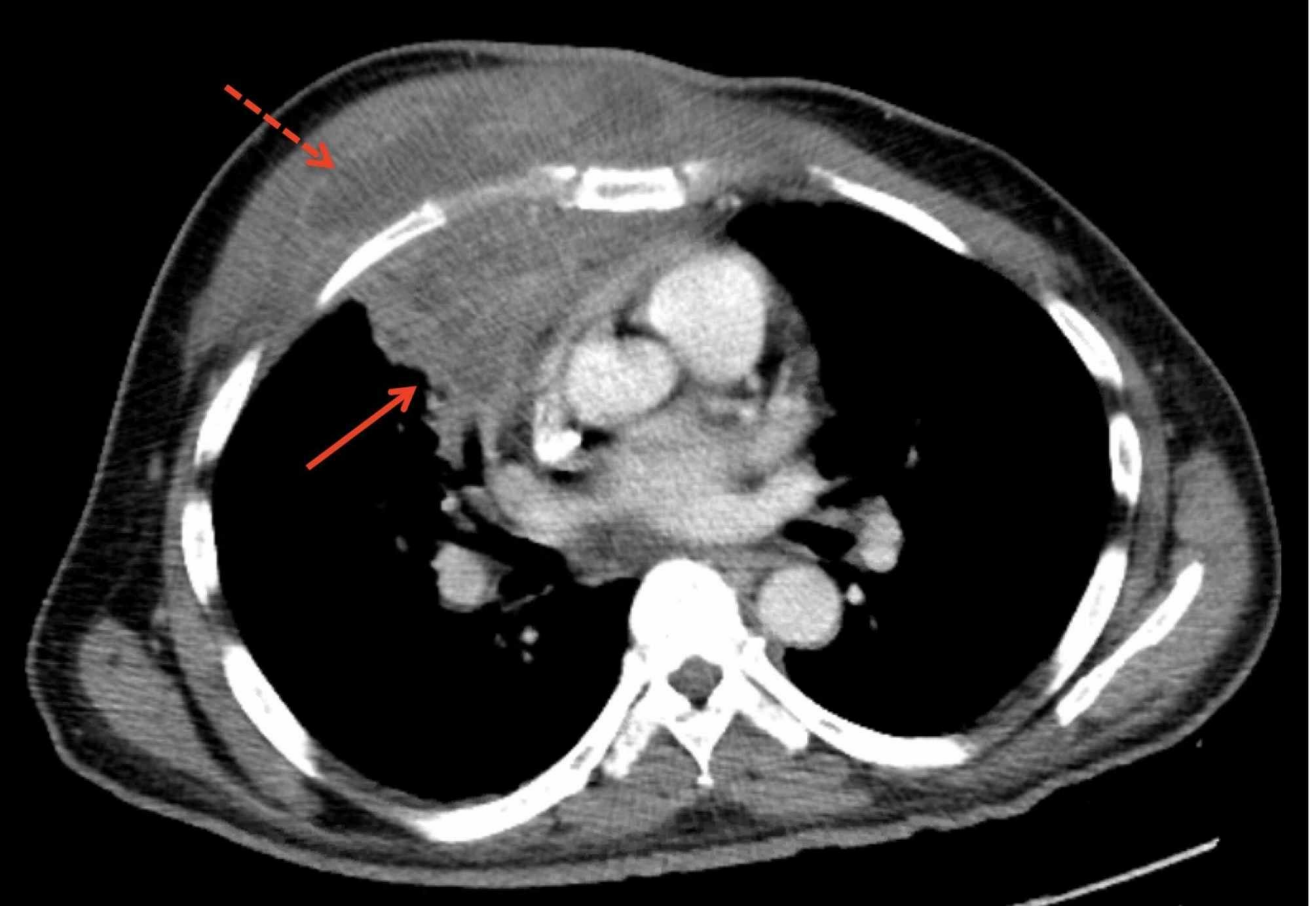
CT with contrast of the chest showing an abscess in the right lung (solid arrow) with extension through the pleura and into the soft tissue and musculature of the right chest wall (dashed arrow).
